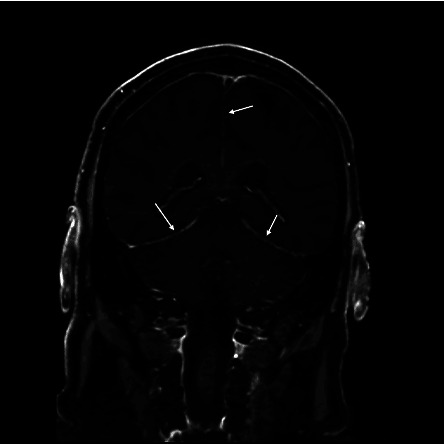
Magnetic resonance image of the brain with intravenous contrast. Postgadolinium T1 with fat saturation series showing diffuse dural thickening and enhancement along the bilateral cerebral hemispheres (arrows).
